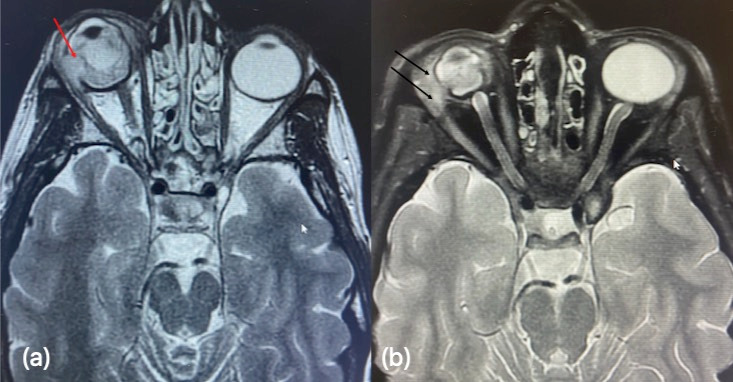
MRI. (a) On the first day of admission, the MRI showed an intraocular inflammatory process affecting mainly the posterior compartment with abundant detritus and retinal and choroidal destructuring. It also showed perforation of the temporal sclera with a parietal defect of 8 mm of maximum anteroposterior axis (red arrow) and discrete intraconal extension of the infectious process with slight edematization of retroocular fat and anterior fibers of the external rectus. Finally, intraorbital periocular purulent tissue was observed extending to the internal canthus. (b) A month and a half after admission, MRI showed collapse of the right eyeball with concentric thickening of its coverings without purulent signal. On the inferolateral border of the orbit, in an extraconal situation, between the internal rectum and lateral rectum, there were two collections of abscess semiology (*black arrows*)
